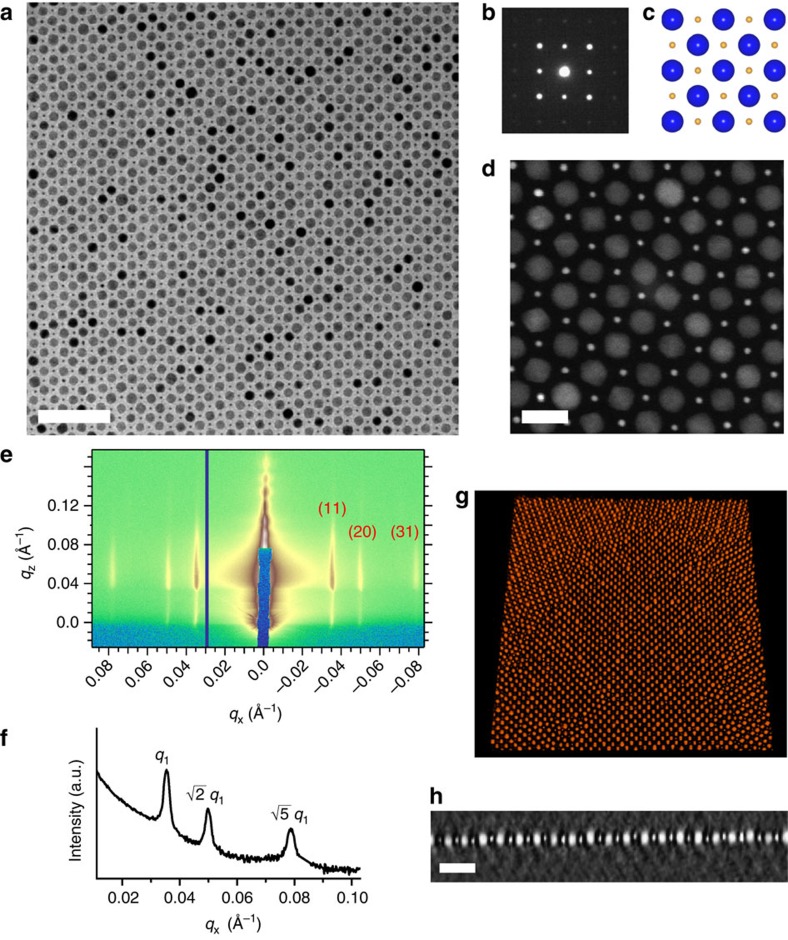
Two-dimensional BNSLs self-assembled from 3.8 nm (3.0 k) Au and 13.4 nm (5.3 k) Fe_3_O_4_ NCs. (a) Low-magnification TEM image and (b) corresponding SAED pattern, (d) HAADF-STEM image of AB-type 2D BNSLs. (c) Structural model of AB-type 2D BNSLs. (e) GISAXS pattern for AB-type 2D BNSLs taken at an incidence angle of 0.2° with 10 keV X-rays. (f) Horizontal line profile of the GISAXS pattern along *q*_*z*_=0.047±0.003 Å^−1^. (g,h) HAADF-STEM tomographic reconstruction of AB-type 2D BNSLs; g is a perspective image of a 3D representation of a subset of the reconstruction and h is a cross-sectional slice showing that the NCs composing the 2D BNSL are coplanar. Scale bars, (a) 100 nm; (d) 20 nm; (h) 50 nm.
